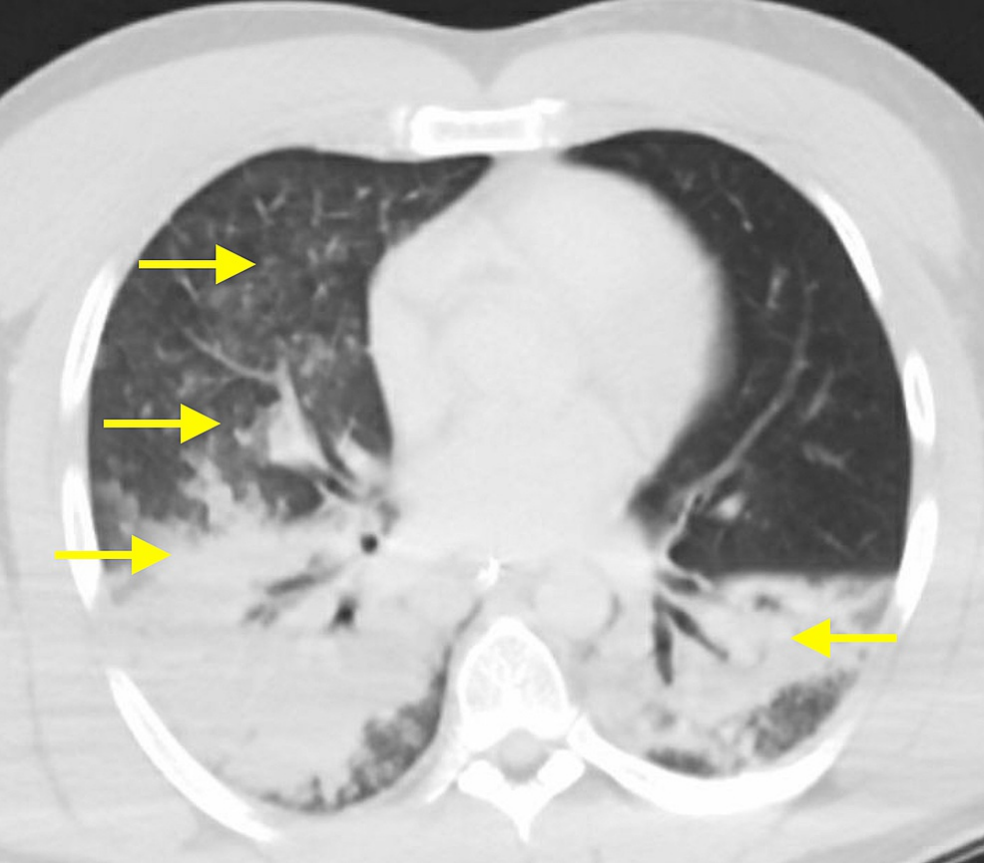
Chest CT scan reveals lung changes characteristic of COVID-19. CT: computed tomography; COVID-19: coronavirus disease 2019
